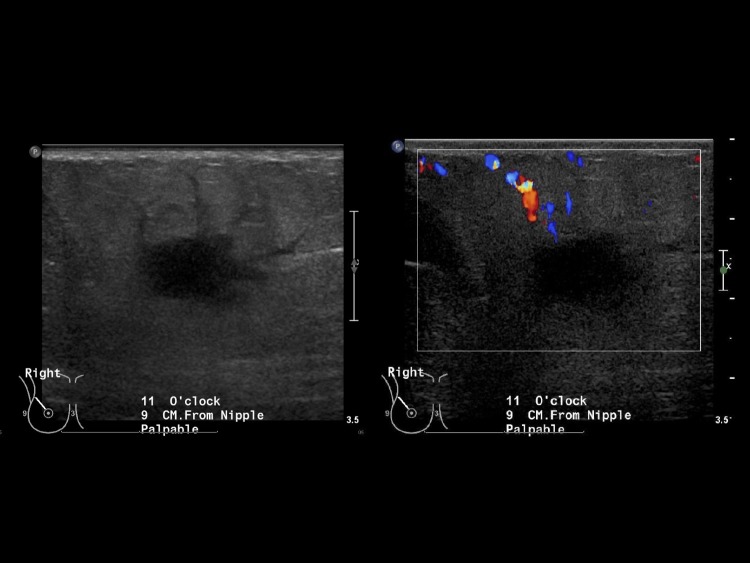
Grayscale and color ultrasound images demonstrate a solid mass with an irregular shape and surrounding edema at site of mammographic and clinically palpable mass, 11 o’clock 9 cm from the nipple.
